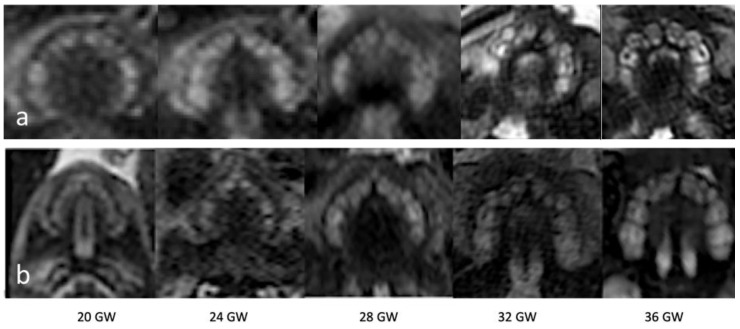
T2-weighted ssFSE images performed with a 3T MRI (a) and with an 1.5T MRI (b) of the dental lamina from GW 20–36, showing a gradual increase in tooth bud visibility, with all tooth buds identifiable at GW 36.
